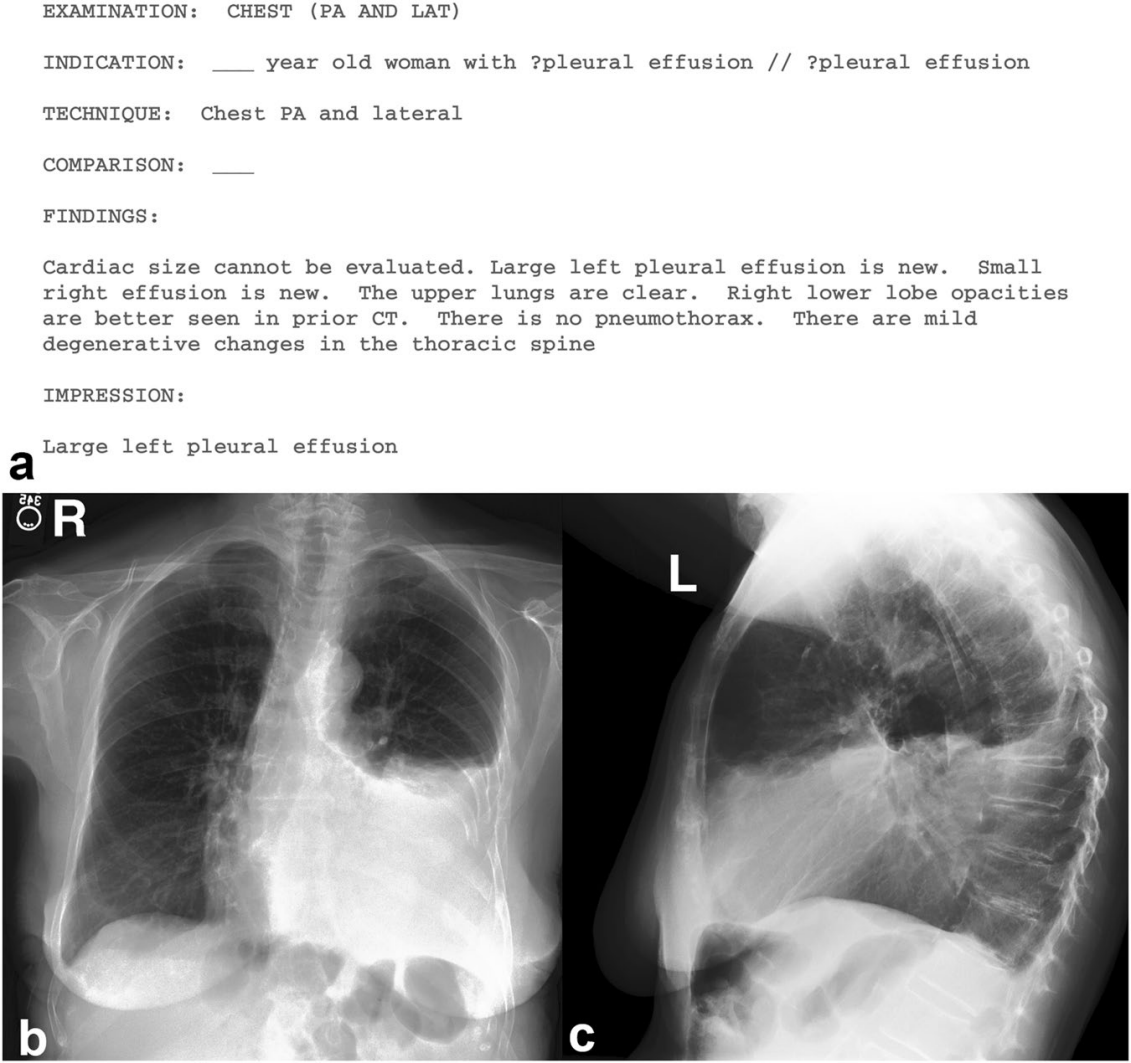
Example study contained in MIMIC-CXR. Above (a), the radiology report provides the interpretation of the image. PHI has been removed and replaced with three underscores (_ _ _). Below, the two chest radiographs for this study are shown: (b) the frontal view (left image) and (c) the lateral view (right image).
